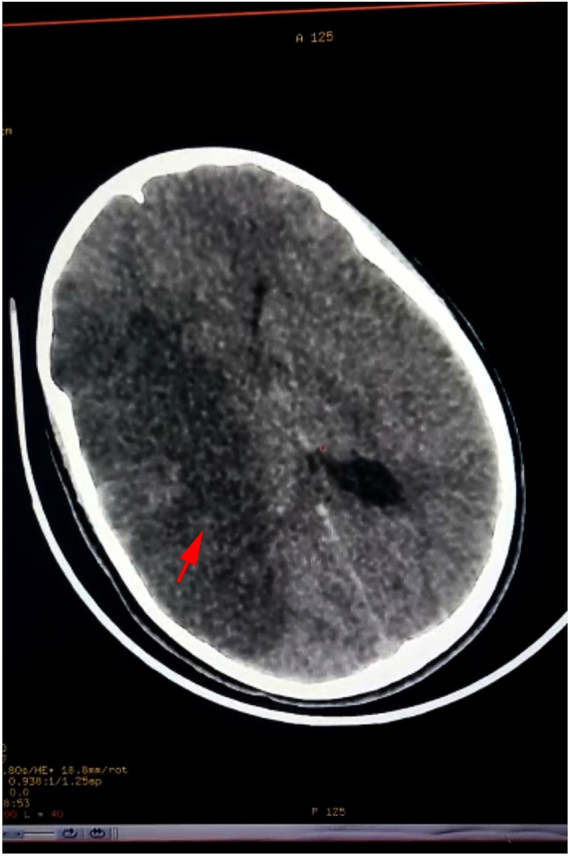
Non-contrast head CT scan revealed a spontaneous hypodensity (arrow) in the right middle cerebral artery territory.
